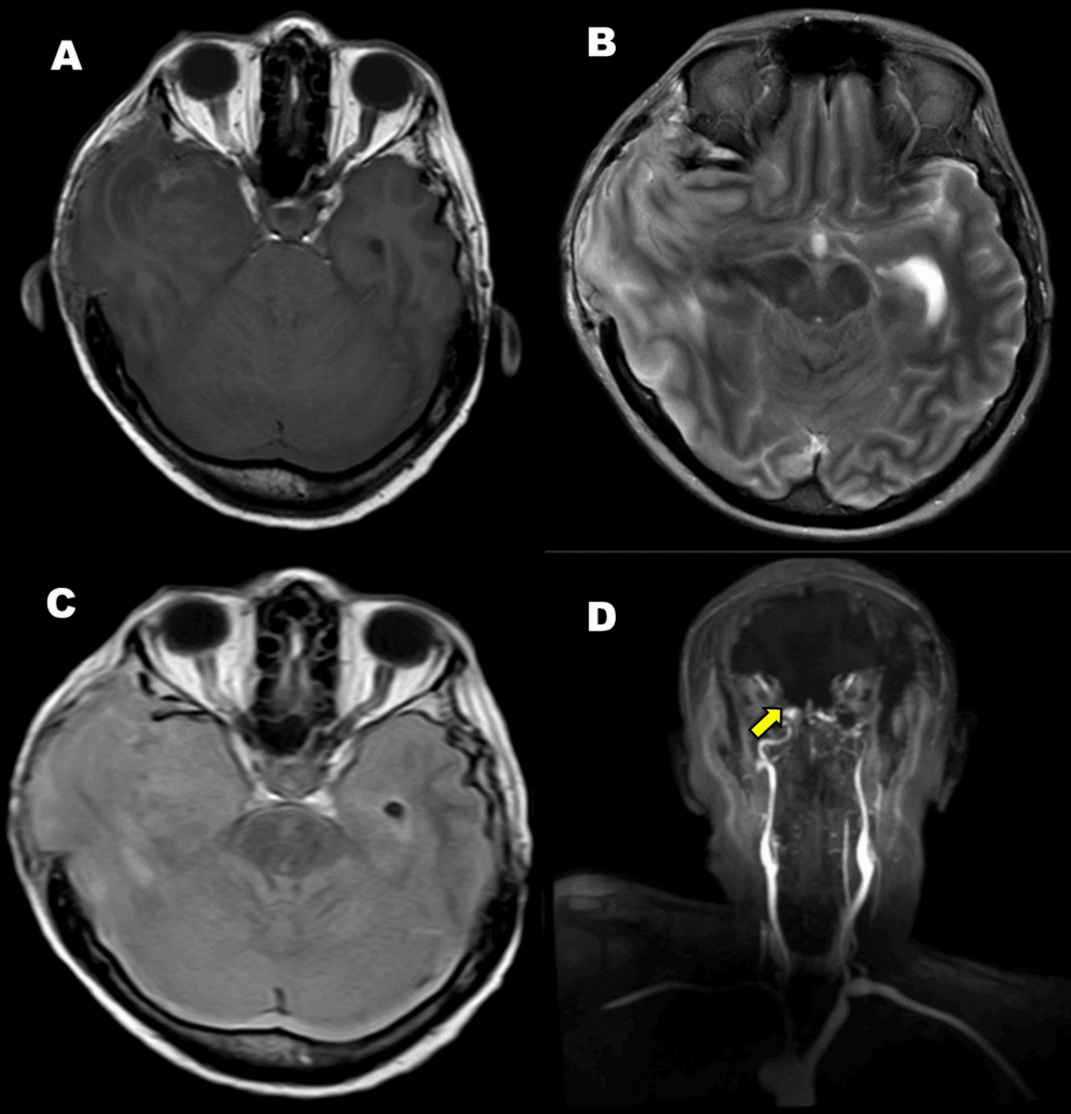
Brain MR angiography. Right frontotemporal craniectomy resulting in transcalvarial herniation. On T1 (A), T2 (B), and FLAIR (C) sequences, diffuse cerebral edema is noted, obliterating subarachnoid spaces in cortical sulci, fissures, and basal cisterns. Additionally, there is absence of vascular (arrow) flow in the vertebrobasilar system, circle of Willis arteries, and middle cerebral arteries, consistent with imaging signs of brain death (D) MR: magnetic resonance; FLAIR: fluid-attenuated inversion recovery
